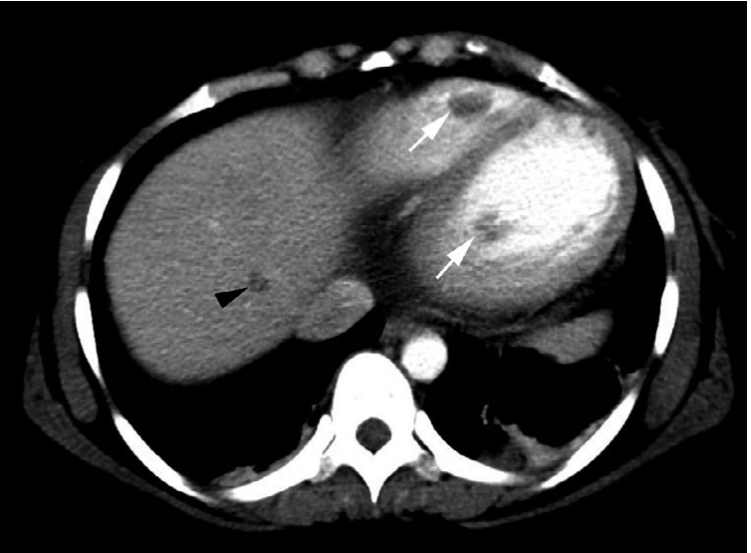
Computed tomography scan showing biventricular thrombi (white arrow) and a low attenuation lesion in the right lobe of the liver (black arrow).
